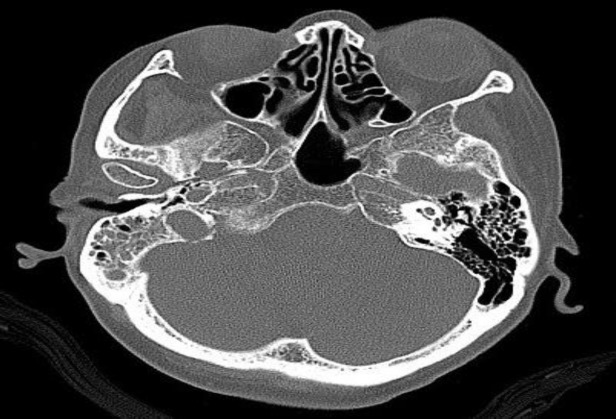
CT temporal bone showing lesions in the mastoid with sclerosis in the basiocciput
